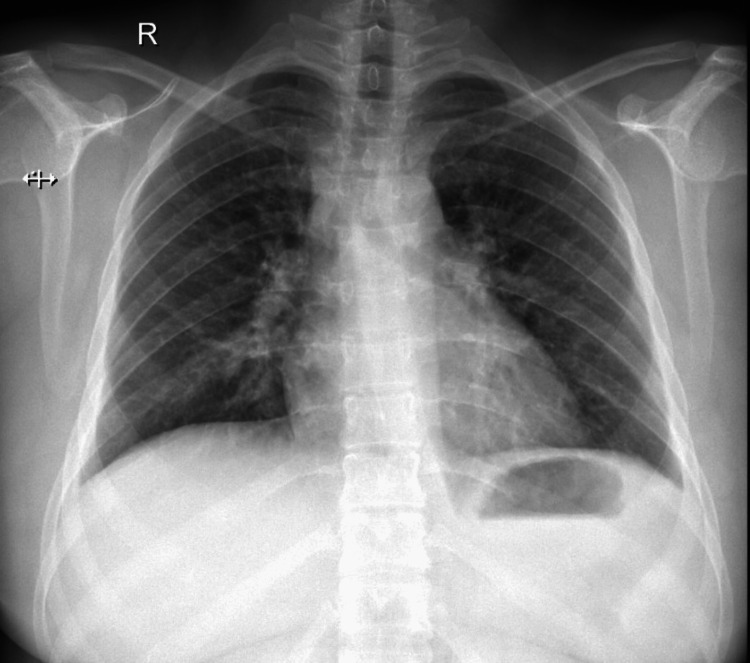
Plain chest radiograph with no apparent abnormalities
